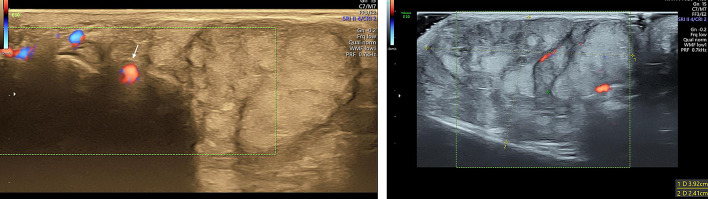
a and b Doppler ultrasound with power doppler shows minimal vascularity within the Mass (M). The 2nd interdigital artery (arrow) is seen away from the mass
